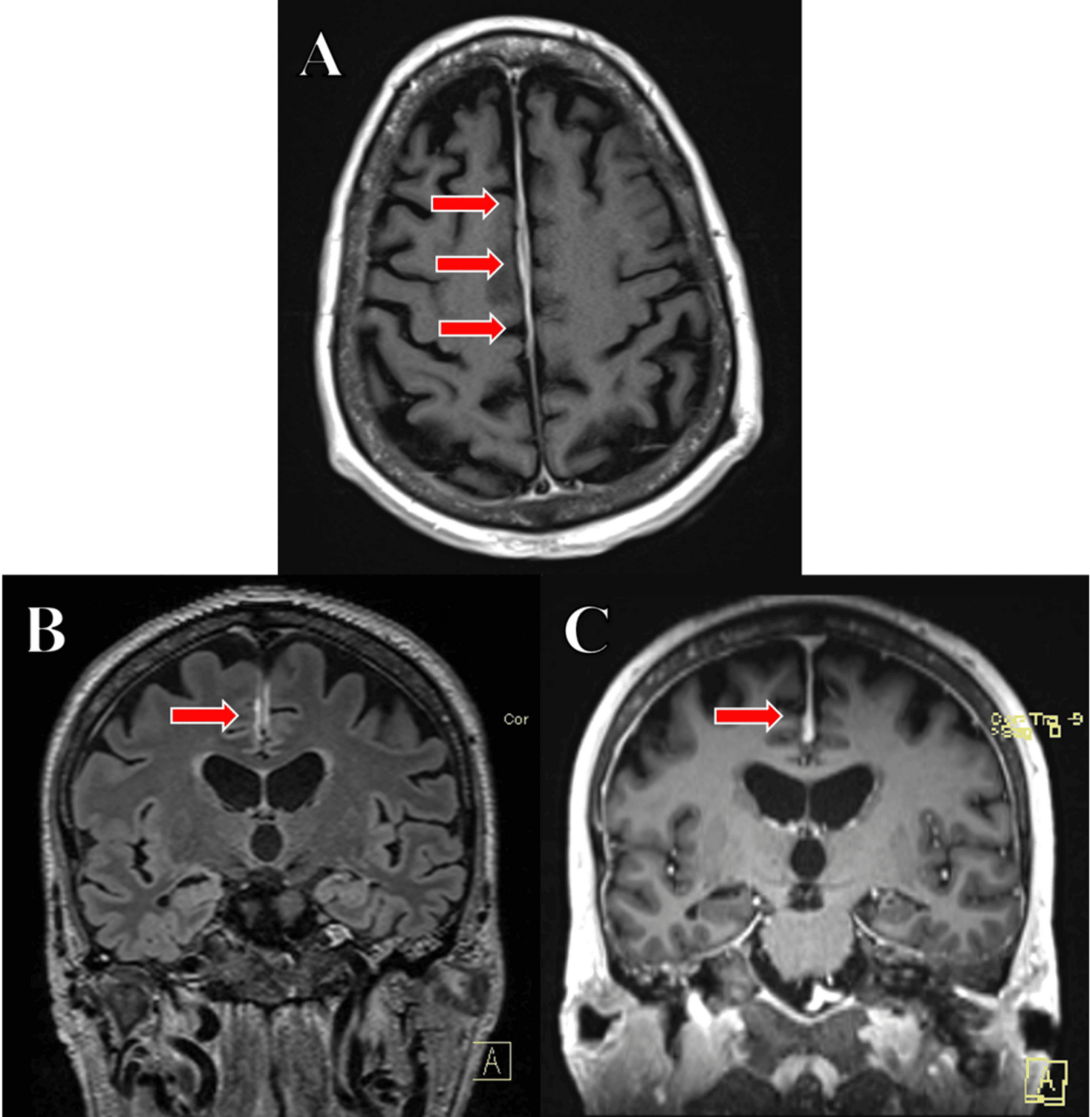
(A, top middle, red arrows) T1 post-contrast axial view showing smooth pachymeningeal enhancement along the bilateral cerebral convexities. (B, bottom left, red arrow) FLAIR coronal view revealing mild nodular dural enhancement along the mid falx. (C, bottom right, red arrow) Post-contrast coronal view demonstrating mild nodular dural enhancement along the mid falx.
